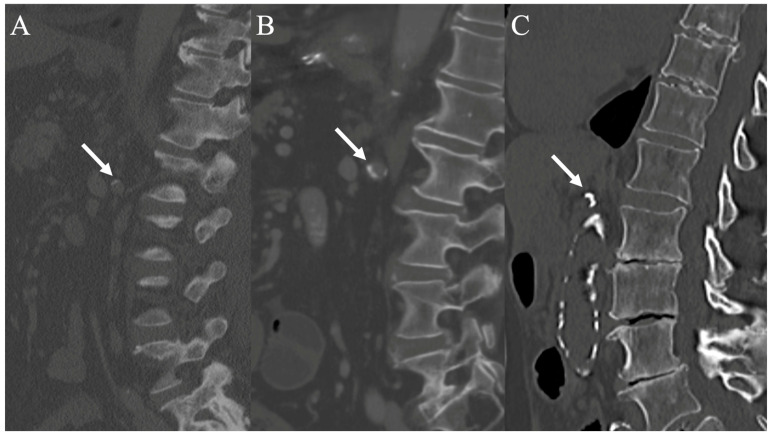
CT scans show the renal artery ostium region in the sagittal plane to assess the morphology and circumference of calcifications. (A) shows thin linear (eggshell-type) calcifications (score 1), (B) shows thick linear calcifications (score 2), and (C) shows bulky calcifications (score 3) (white arrows).
